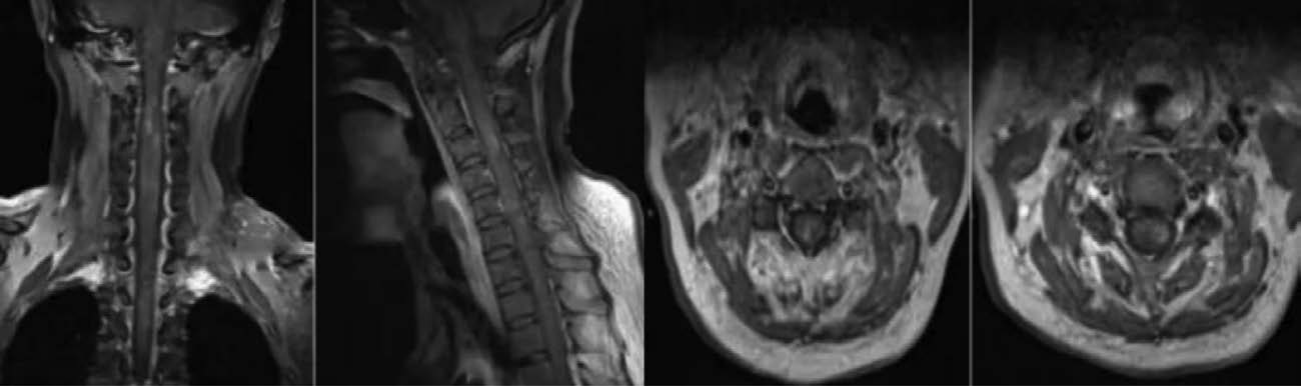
MRI of the cervical spine showed significant signal changes with enhanced and patchy C2-5 levels.
